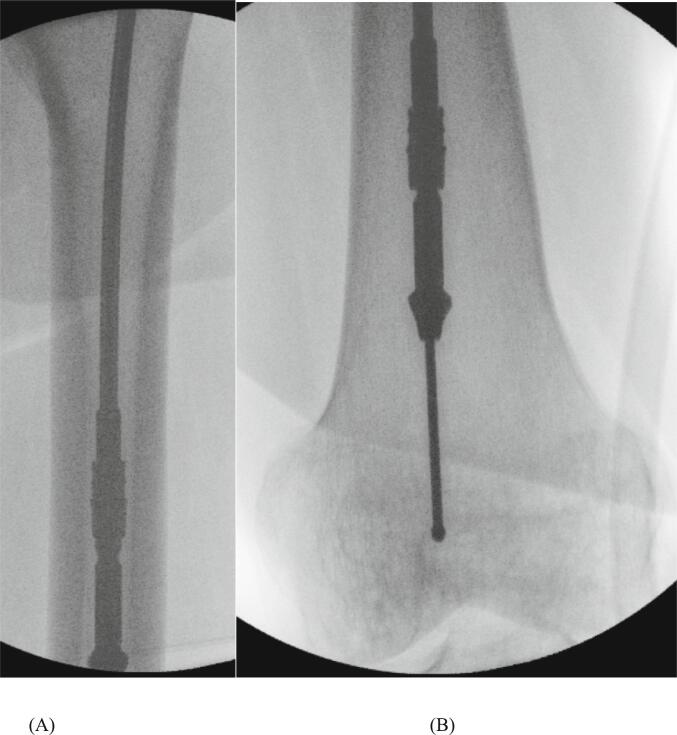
Intraoperative radiographs of reaming at the shaft (A) and at the intercondylar region (B) using the Reamer-Irrigator-Aspirator system.
